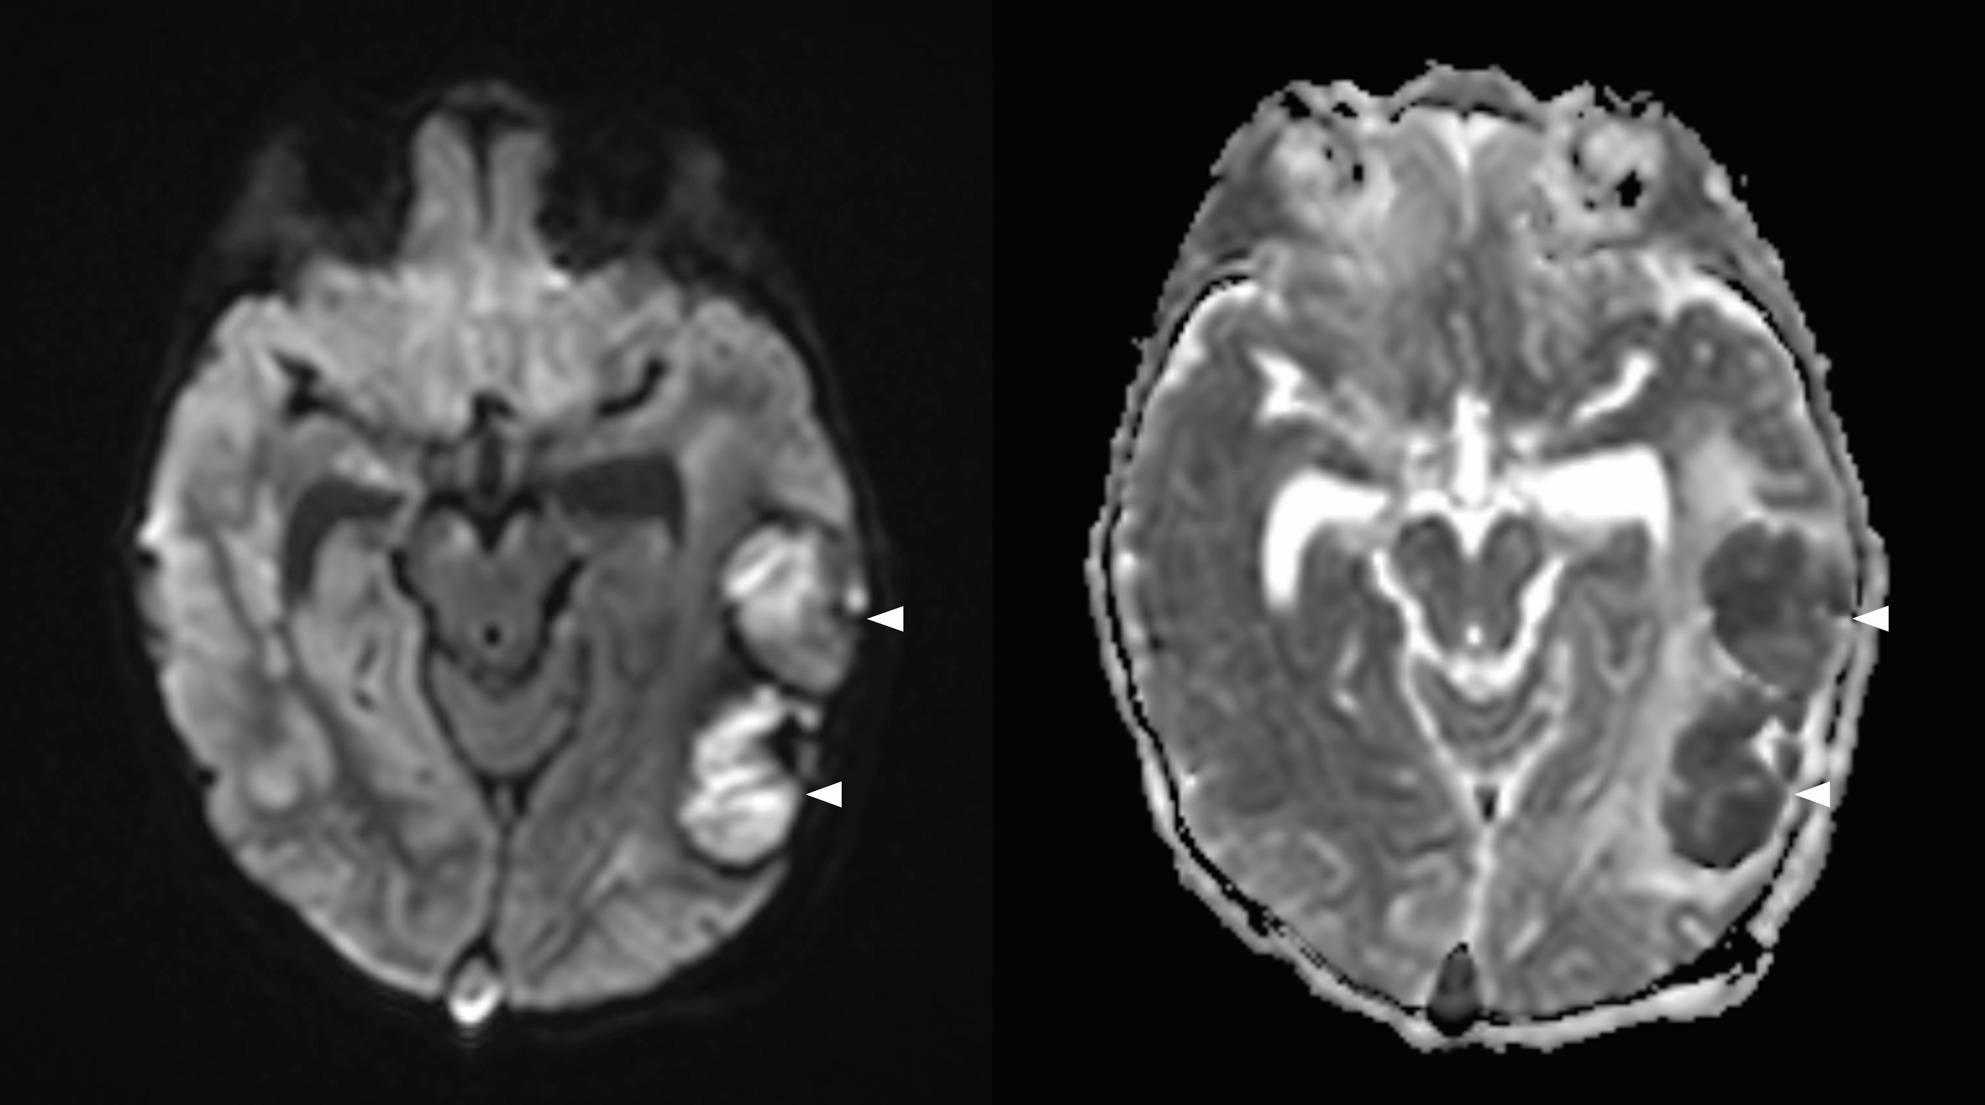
Diffusion-weighted image (DWI) and the corresponding ADC map reveal multiple areas of diffusion restriction consistent with parenchymal infiltration. Cytotoxic edema in the left posterior temporal region (arrowheads), likely representing acute Labbe venous thrombosis, is also present
